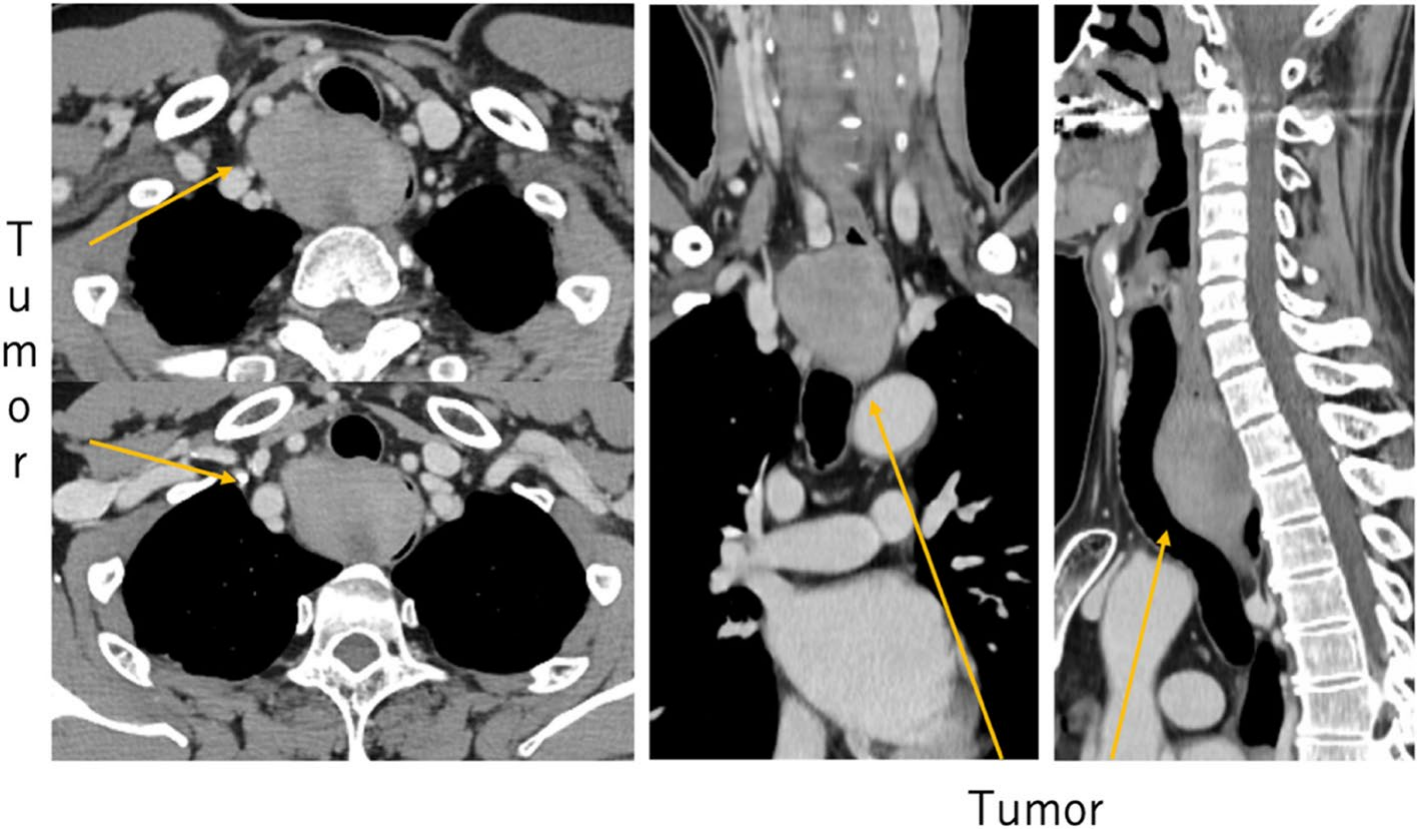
A contrast-enhanced CT scan revealed a uniformly enhanced 5 × 3.5 × 5.4 cm tumor without invasion to other organs or enlarged surrounding lymph nodes
